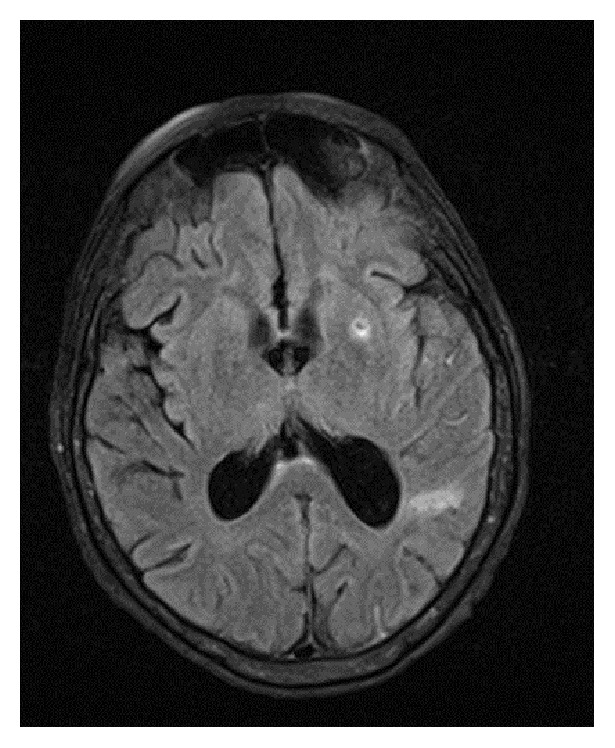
MRI of the brain demonstrating punctate areas of intraparenchymal hemorrhage.
